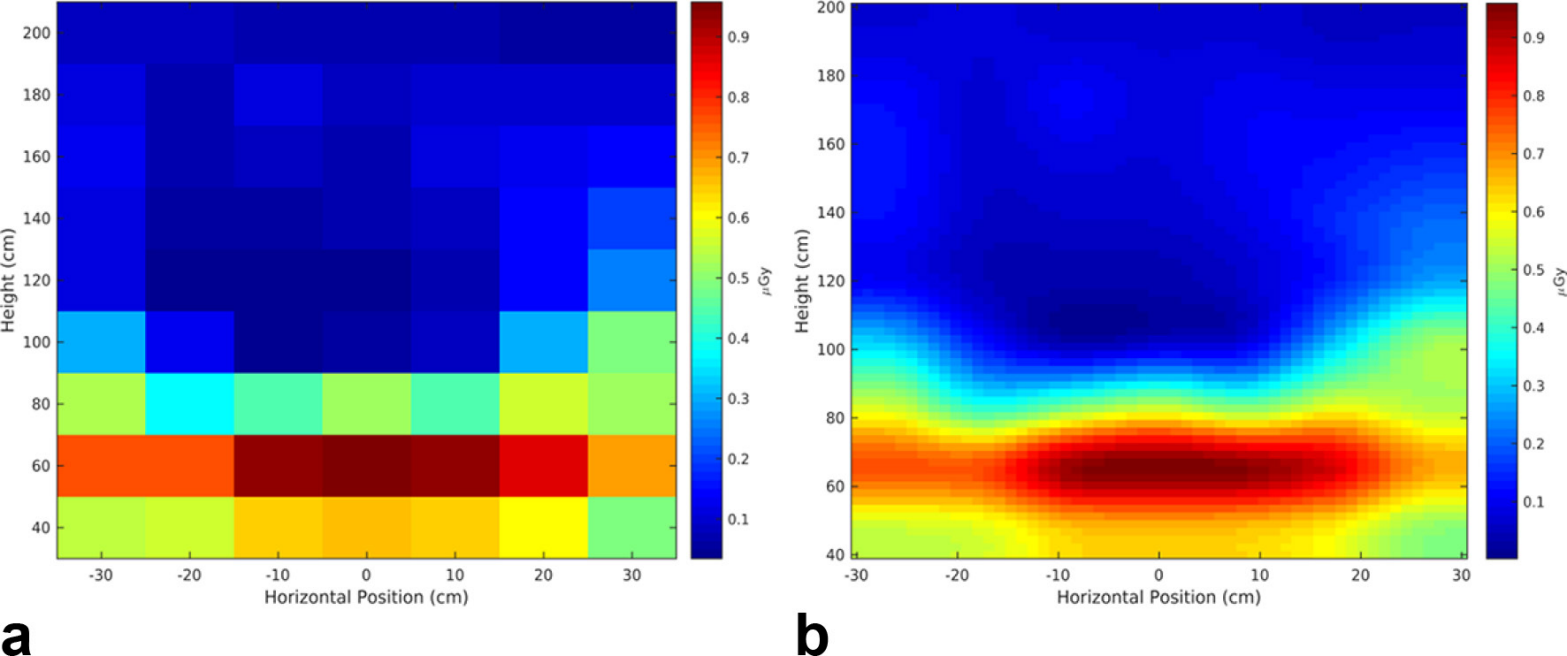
(a) Mapping of the measured stray radiation air kerma values for a hand-held dental X-ray device positioned for a bitewing image of the molars, indicating the spatial variation for each of the measurements. (b) For visual purposes only, a grid of more refined pixels of the measured stray radiation values generated by a bicubic interpolation using a 4 × 4 neighbourhood weighted average (MathWorks, Natick, MA). Note that any calculations used throughout this work were performed with measured values only, and not interpolated values.
